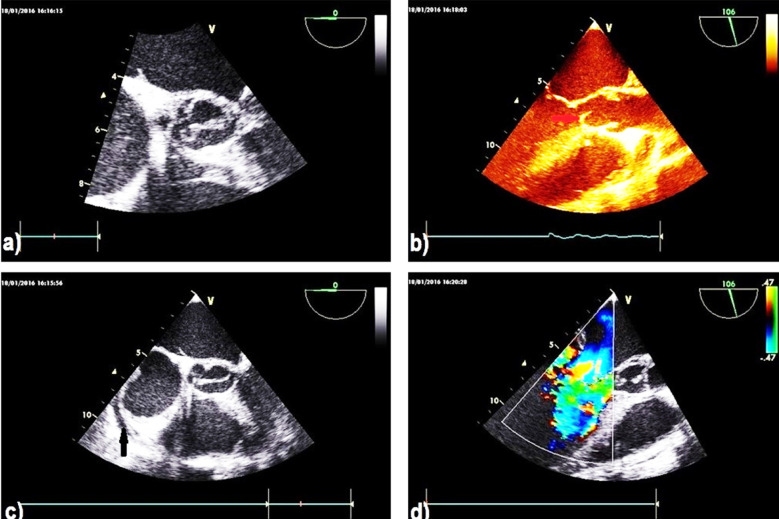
Trans esophageal echocardiography (TEE) show a) detracted aortic valve, b) the presence of sub aortic membrane (arrow), c) minimal pericardial effusion (arrow), d) severe mitral regurgitation (MR) with perforation of the anterior mitral valve leaflet.
